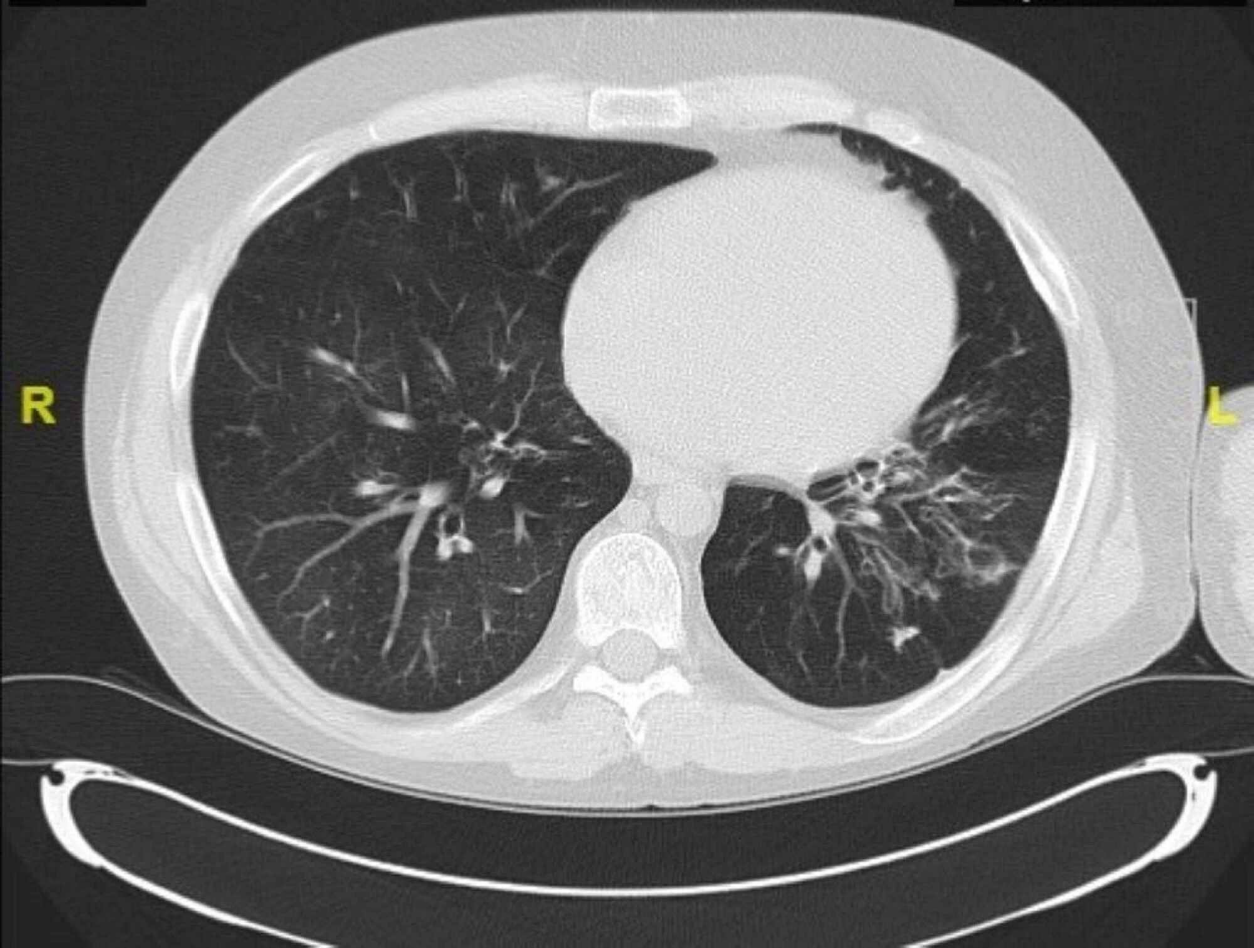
CT chest 2.5 years after initial admission CT chest with findings of permanent cavitary lesions.
